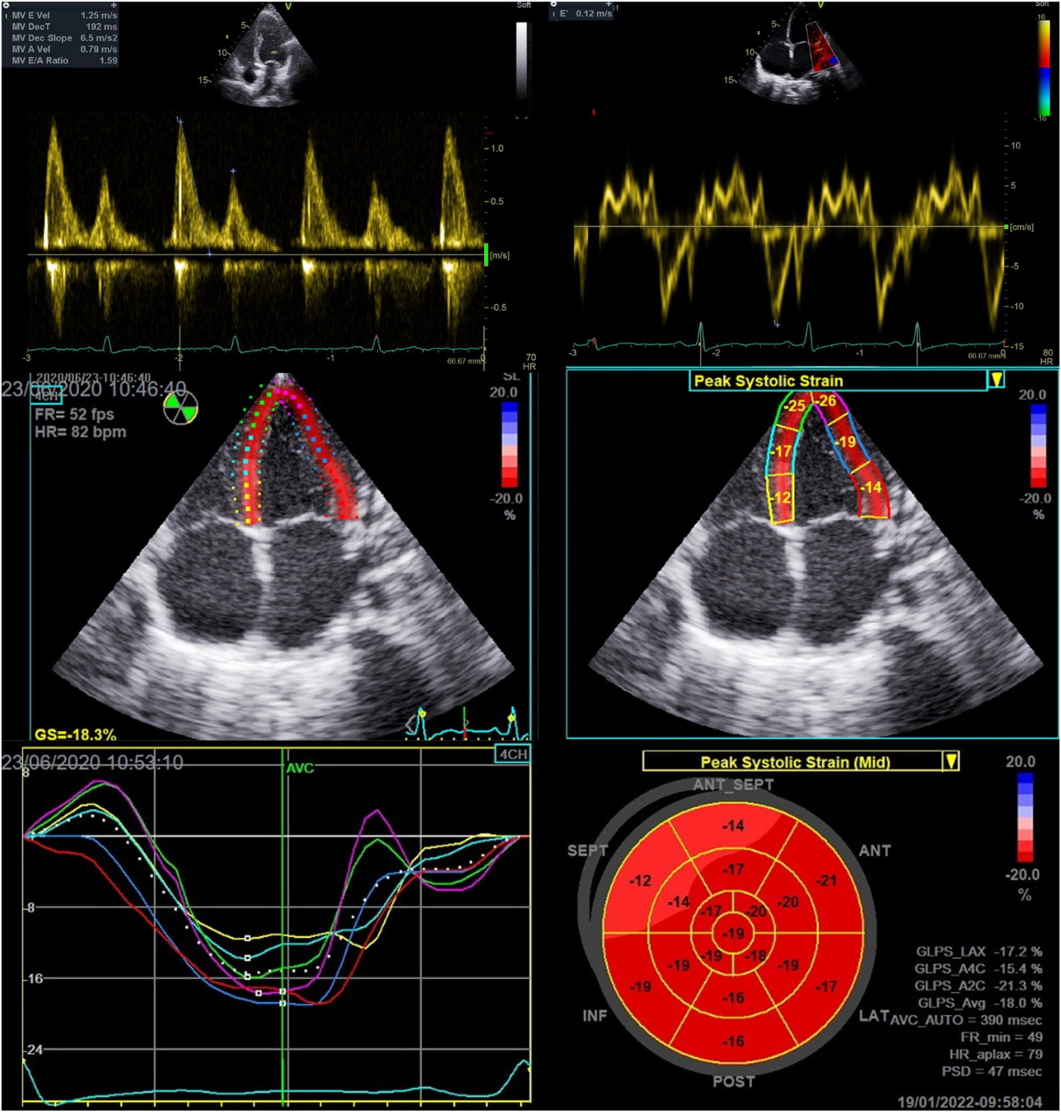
Doppler and strain echocardiographic images. *Top left*, pulsed-wave doppler recording at mitral inflow showing E, A velocities and E-wave DT. *Top right*, tissue doppler imaging at lateral mitral annulus showing e’. *Mid left*, region of interest tracing in the apical 4-chamber view during offline analysis using semi-automatic speckle-tracking software. *Mid right*, segments of left ventricle as seen in the apical 4-chamber view. *Bottom left*, peak systolic strain waveform obtained from apical 4-chamber view shown in mid panel. *Bottom right*, Bull's eye plot of longitudinal strain 3 apical views showing strain values of each segment
